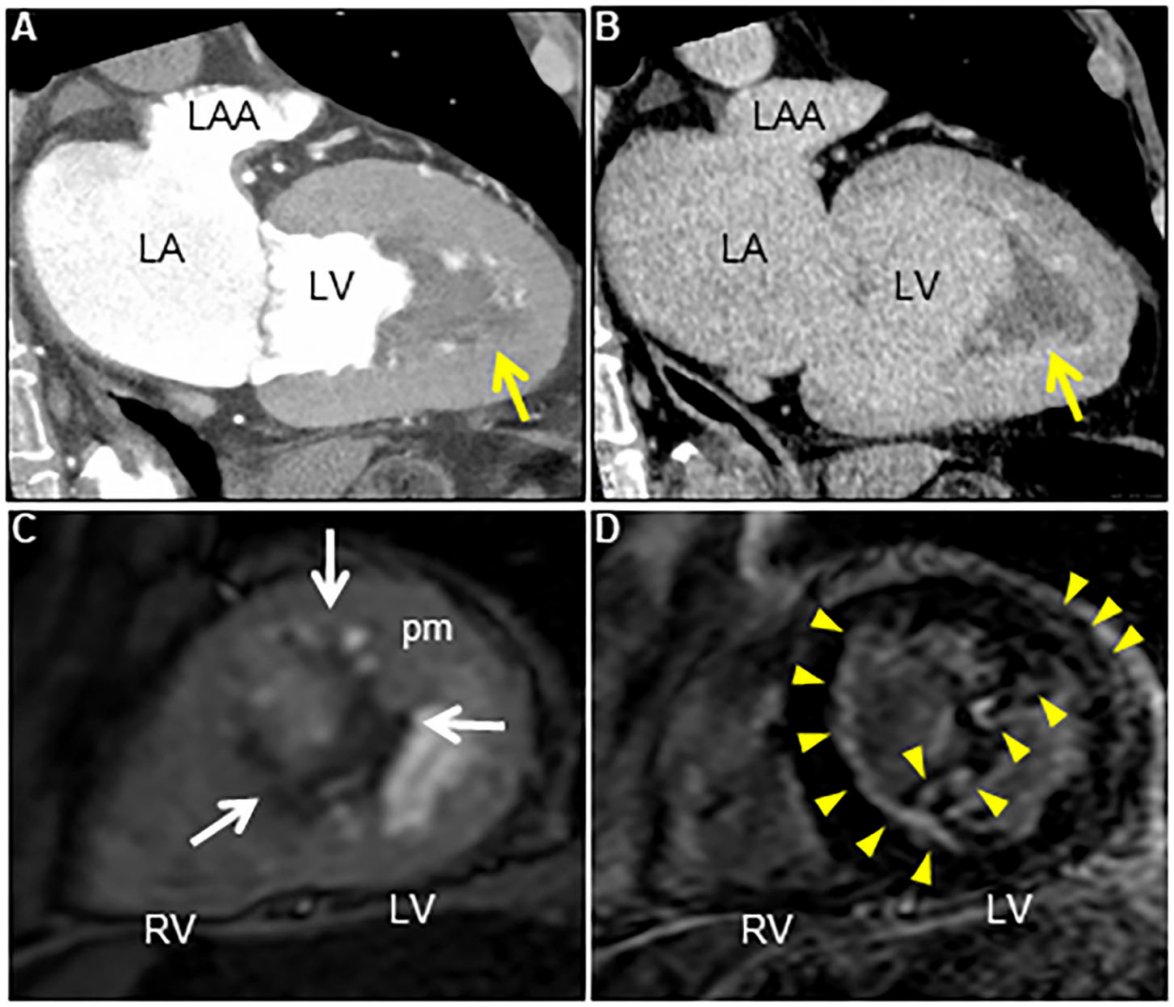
(A) Contrast-enhanced computed tomography scan at early phase reveals an irregular cardiac mass attached to the left ventricle in several locations (arrow). (B) Note the reduced mass with a delayed contrast enhancement at late phase (arrow). (C) Axial dynamic cardiac magnetic resonance imaging reveals a low echogenic left ventricular mass attached to the anteroseptal and inferior walls, and the anterolateral papillary muscle (arrows). (D) Late gadolinium enhancement image reveals subendocardial enhancement in anteroseptal and inferior walls, mid-wall enhancement in lateral wall, and heterogeneous enhancement within the cardiac mass and the adjacent anterolateral papillary muscle (arrowheads). LA, Left atrium; LAA, Left atrial appendage; LV, Left ventricle; pm, Papillary muscle; RV, Right ventricle.
